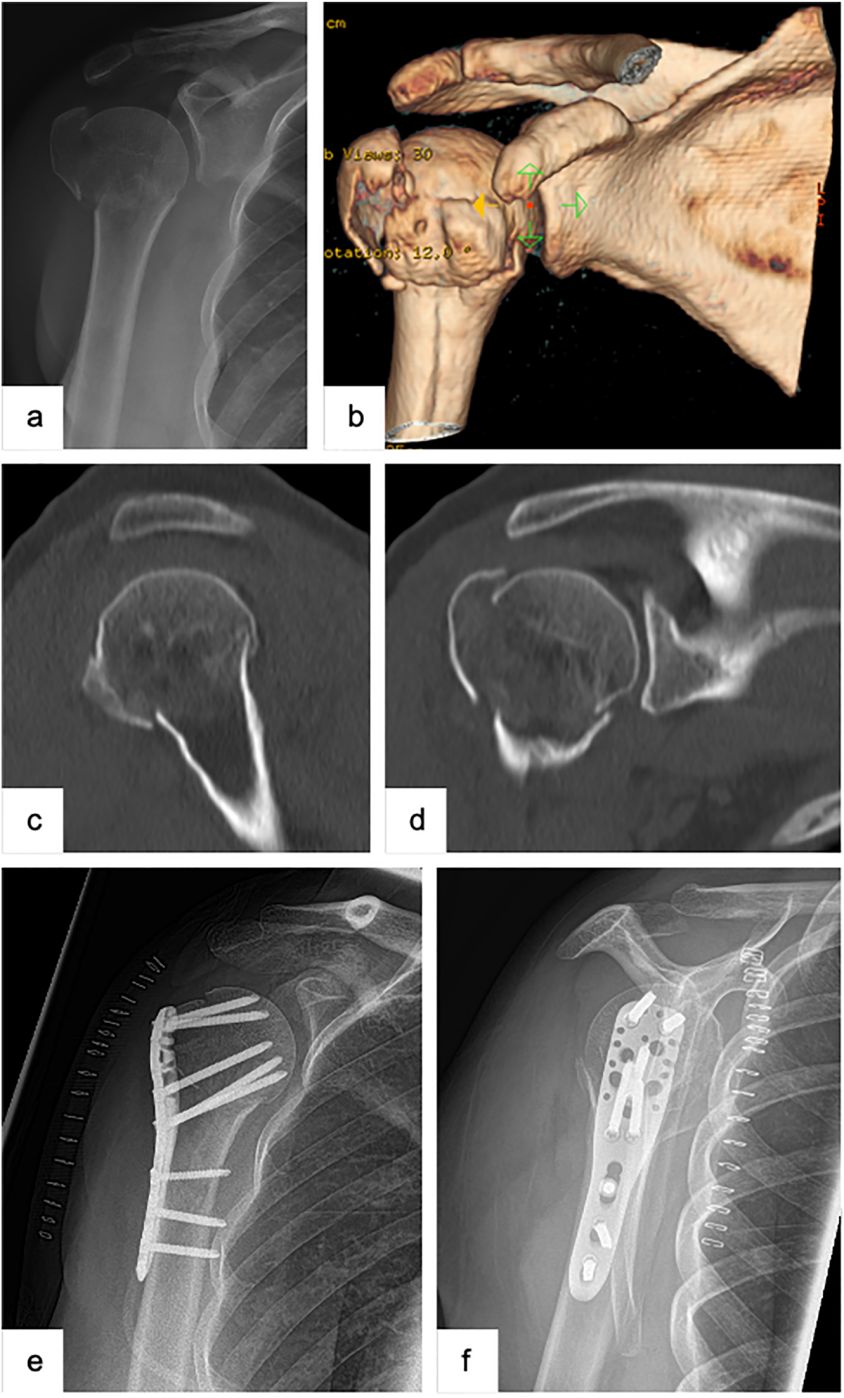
Female patient (53 y), right shoulder, valgus impacted, dislocated 3-part proximal humerus fracture (preoperative X-ray and (3D-) CT-scan: a – a.p., b – 3D-CT-reconstruction, c – sagittal, d – coronal), open reduction and internal fixation with a locking plate osteosynthesis (postoperative X-ray: e – a.p., f – Y-plane).
